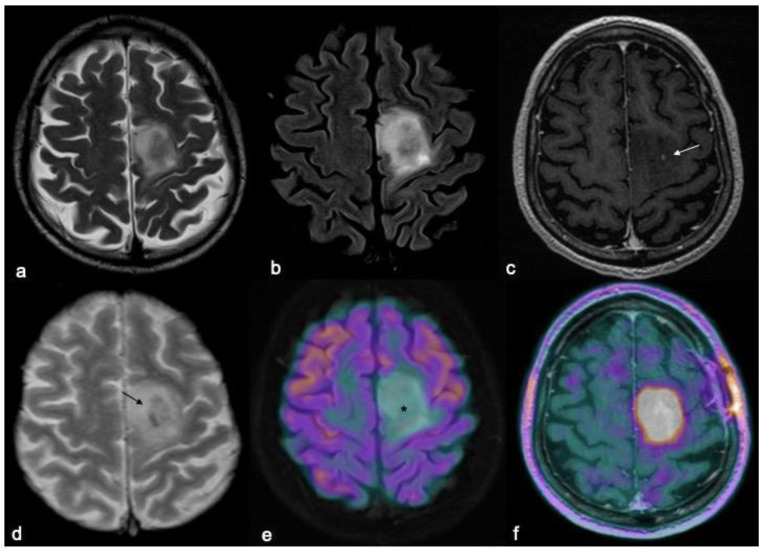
MRI and PET characteristics of a diffuse IDH-MUT 1p/19q codeleted glioma (oligodendroglioma grade 2). T2w fast spin echo (a), T2w FLAIR (b), T1w fast spin echo contrast-enhanced (c), T2*-weighted gradient echo (d), FDG-PET/MRI (e), and DOPA-PET/MRI (f) images are shown. Mesial frontal mass with a low mass effect and without any significant enhancements after contrast (arrow in (c) indicates the only spot of blood–brain barrier leakage), and some intratumoral calcifications (arrow in (d); CT not shown) with no significant radiotracer uptake by FDG-PET (asterisk in (e)), but showing a lively uptake of the PET-DOPA radiotracer (f).
